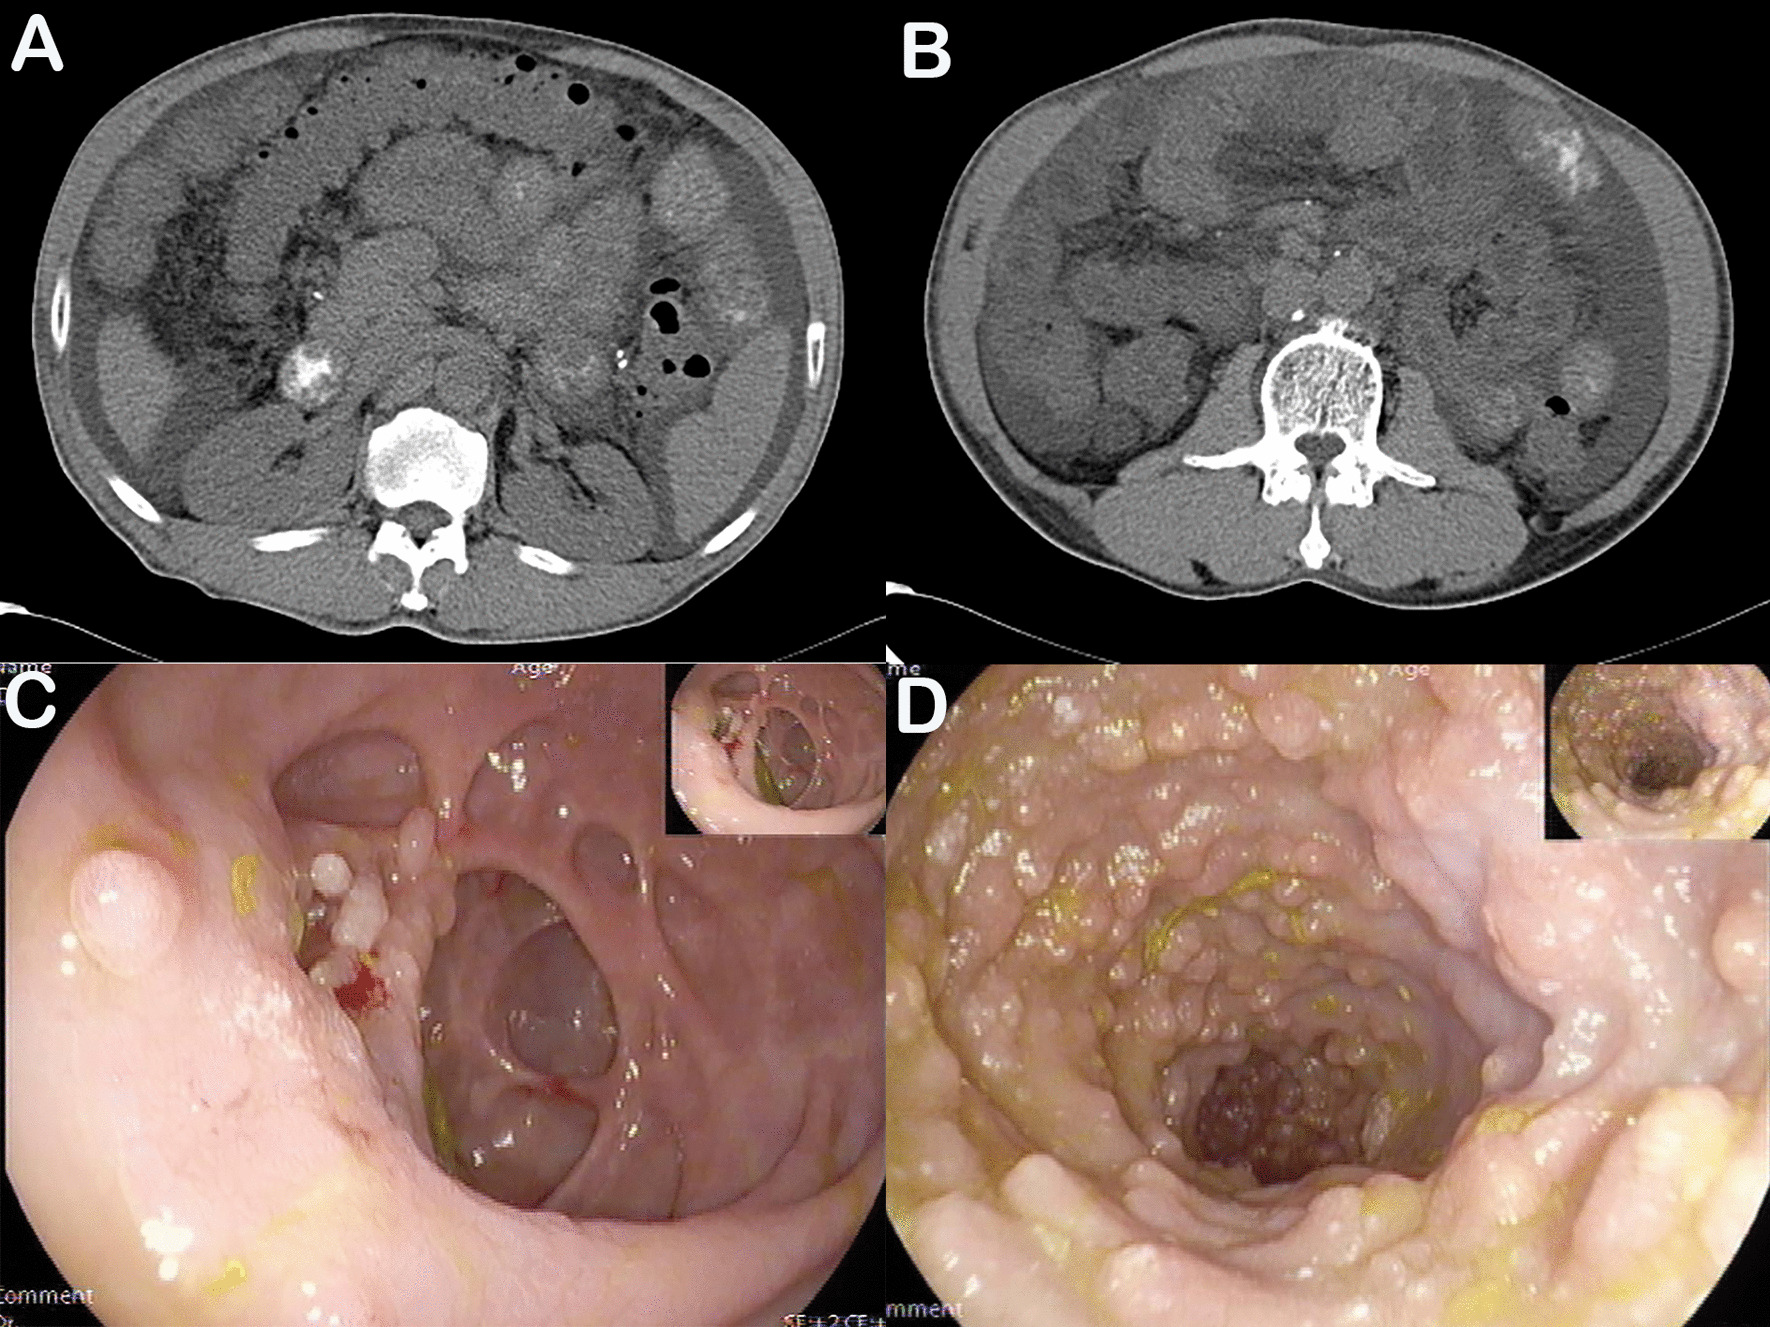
Plain computed tomography shows ascites and diffuse thickening of multiple small bowel loops (a, b). Endoscopic views of the ileocecal valve (c) and terminal ileum (d)
